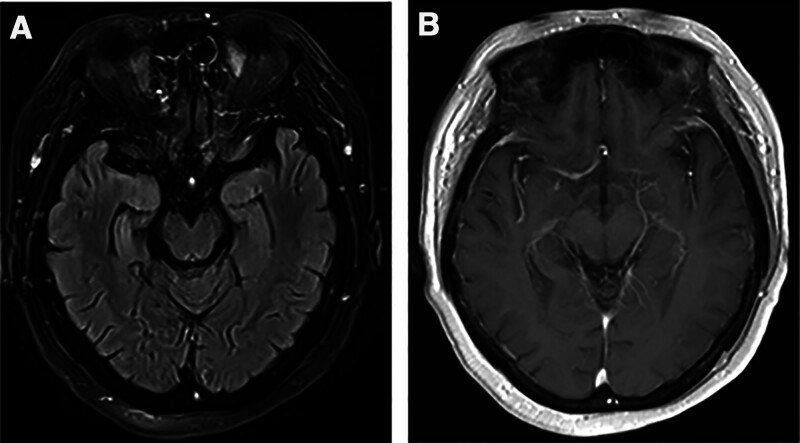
Repeat cranial MRI scan features of the patient after 3 months of efgartigimod treatment. (A) The abnormal signals in the medial aspect of the right temporal-occipital lobe on FLAIR-weighted sequence were further attenuated compared to the second cranial MRI scan. (B) The lesions in the medial aspect of the right temporal-occipital lobe exhibit no contrast enhancement.
